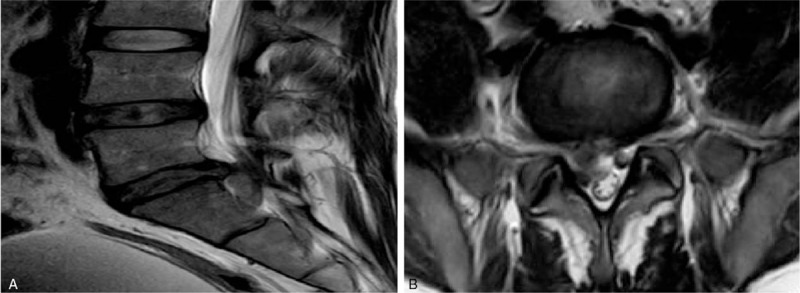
Pre-operative MRI of lumbar disc herniation. (a) Pre-operative MRI of lumbar disc herniation at L5, S1 in sagittal view. (b) Pre-operative MRI of lumbar disc herniation at L5, S1 with compression of a nerve of root left.
